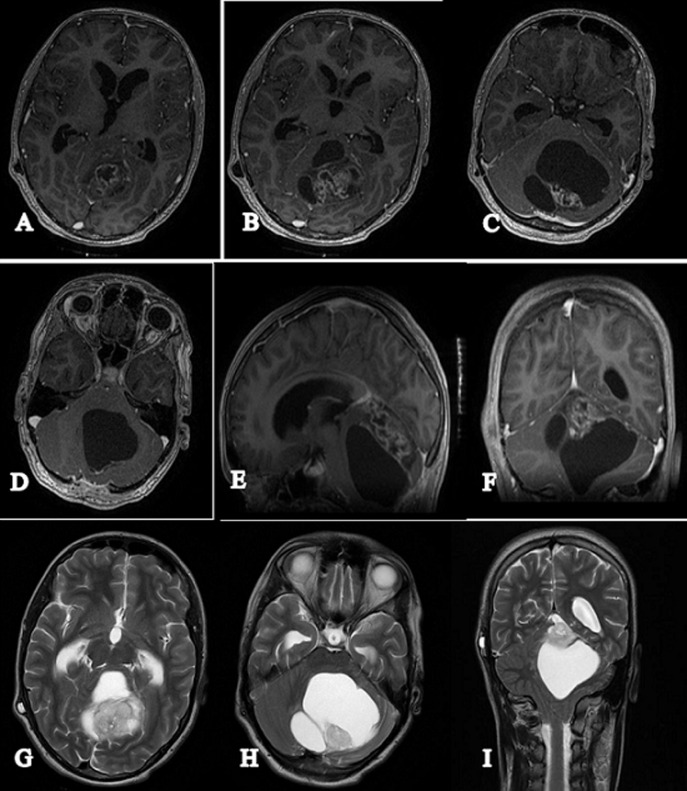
axial section brain MRI T1 injected (A+B+C+D) sagittal slice (E) and coronal slice (F), axial sections T2 (G+H) and coronal slice (I), showing cerebellar hemangioblastoma with both fleshy and cystic components
